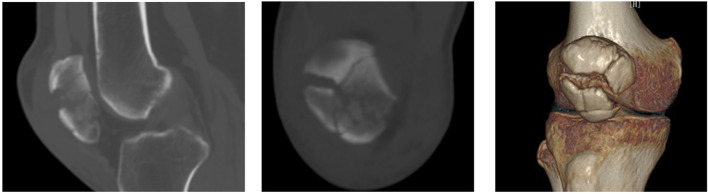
CT imaging of a patellar fracture involving the articular surface and inferior pole of the patella.
